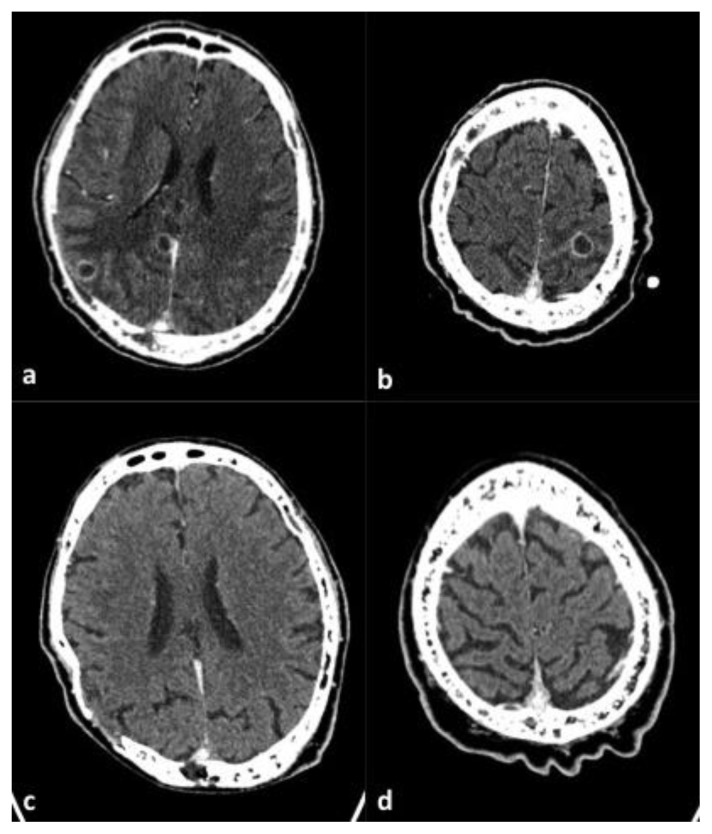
Axial brain CT scan post-contrast medium administration. Images obtained before therapy show two ring-enhancing lesions with abundant peripheral oedema in right parietal lobe (a) and one in left parietal lobe at the vertex (b). Images obtained after therapy (c,d) show the complete resolution of the lesions.
